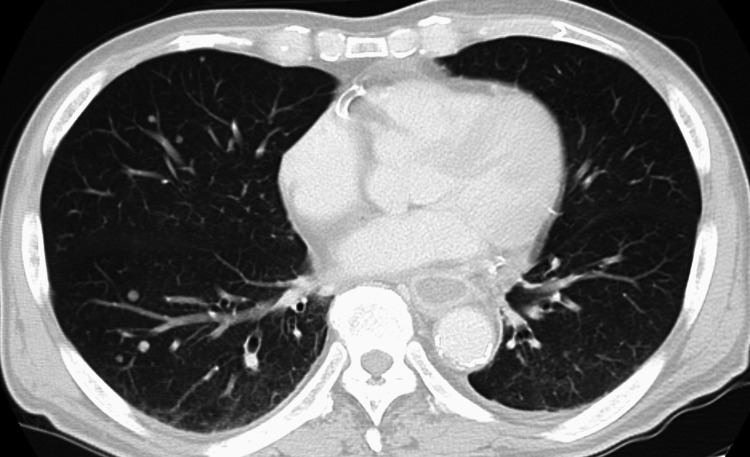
Plain chest CT detecting multiple pulmonary metastases.
